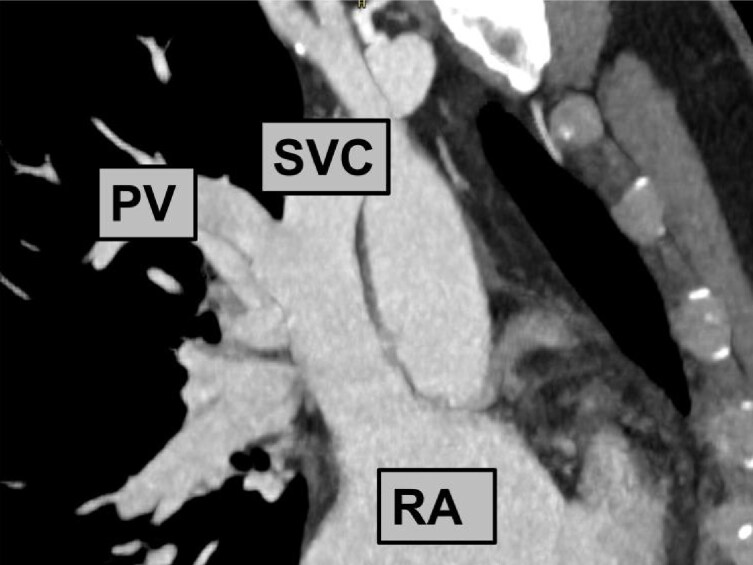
Computed tomography reconstruction of the anatomy (similar view as fluoroscopy in *Figure 3*). PV, pulmonary vein; RA, right atrium; SVC, superior vena cava.
